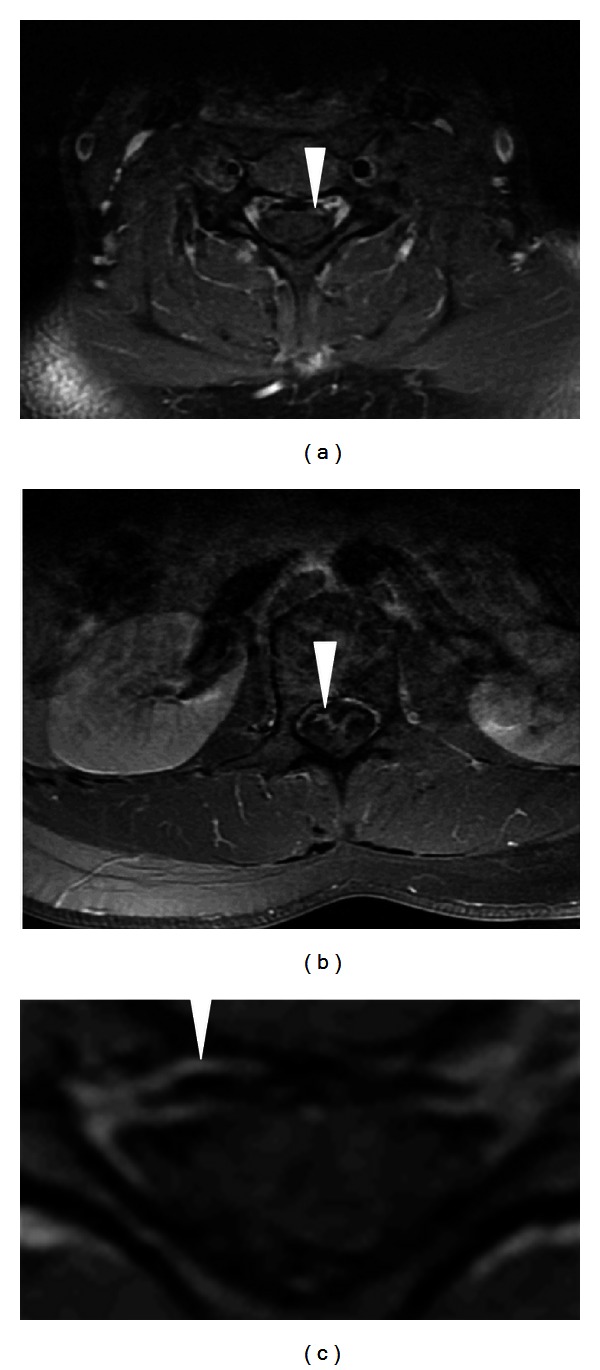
(a) Postgadolinium axial MRI sequences of the cervical cord (a-b), and distal thoracic cord (magnified in (c)) reveals anterior nerve root enhancement consistent with an inflammatory polyneuropathy.
